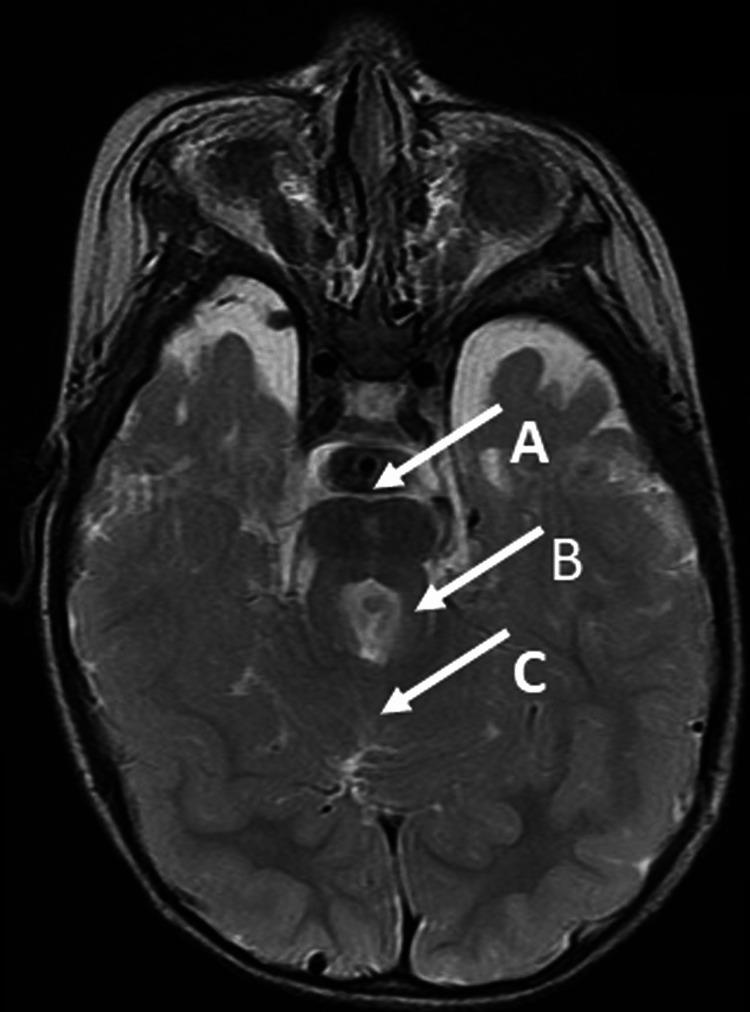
T2-weighted axial images on MRI of child 3 The MRI image was taken when he was 21 months old. The "Molar tooth sign" image is characterised by a deep posterior interpeduncular fossa (white arrow A), thickened and elongated superior cerebellar peduncles (white arrow B), and hypoplasia or agenesis of the cerebellar vermis (white arrow C). MRI: magnetic resonance imaging
